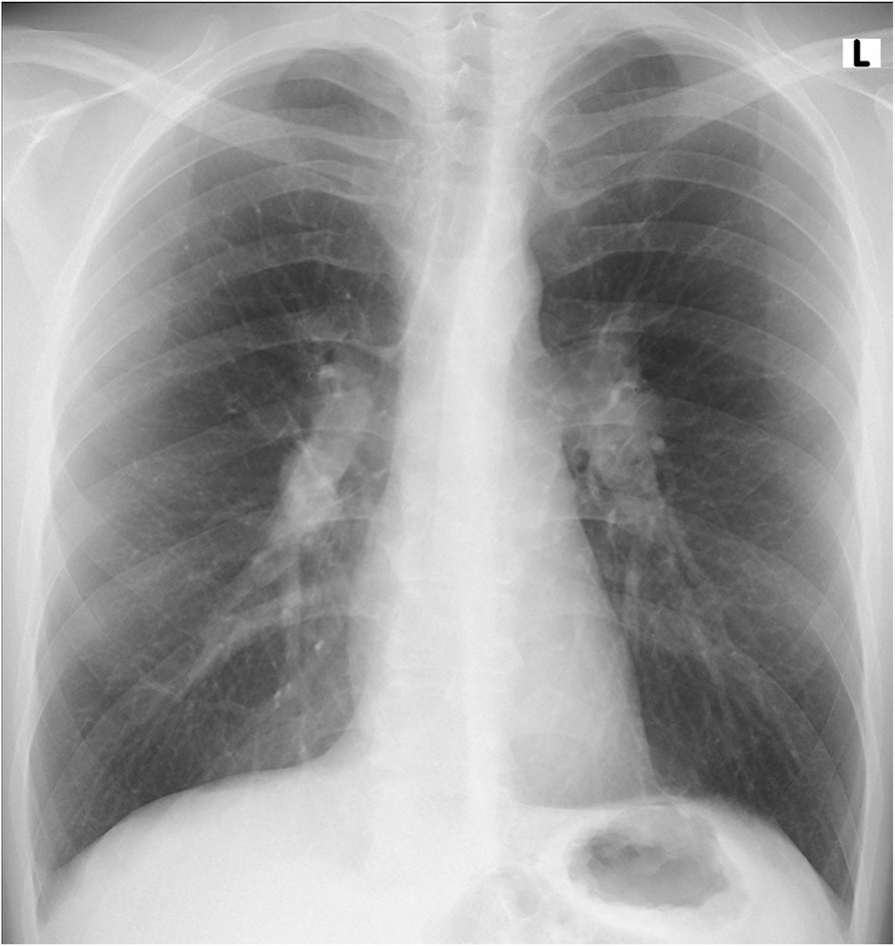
X-ray showing stage I sarcoidosis
